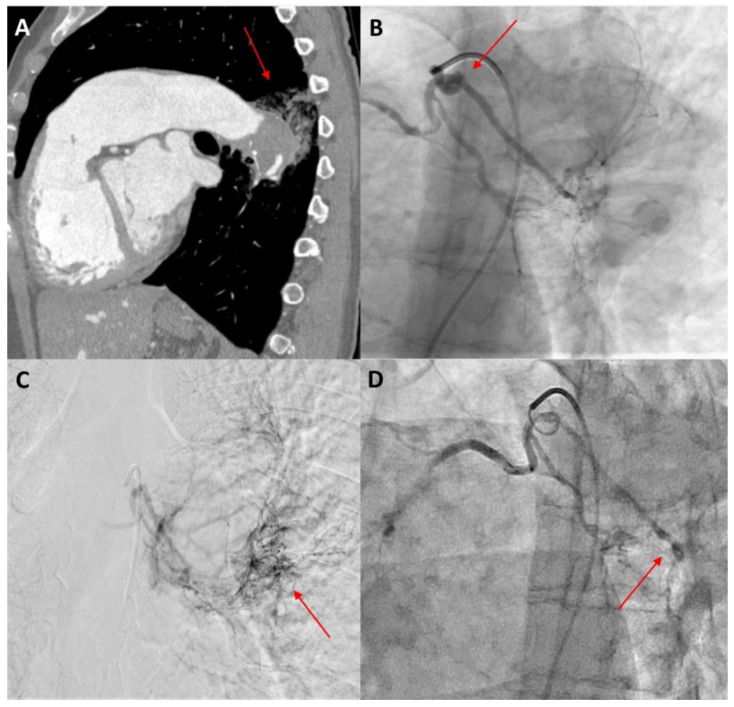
A 40-year-old female patient with Eisenmenger syndrome on the background of a large secundum ASD presenting with recurrent episodes of haemoptysis. (A) Sagittal view of thorax computed tomography after intravenous contrast medium injection, showing aneurysmatic dilatation of the left pulmonary artery with intraluminal thrombus and adjacent post-bleeding infiltrations in the lung parenchyma (red arrow). (B) Catheterization of the bronchial arteries with a 5 Fr Cobra catheter, showing the tortuous origin of the left bronchial artery (red arrow). (C) Angiographic image in a delayed parenchymal phase, showing abnormal imaging of the left posterior lung parenchyma adjacent to the descending aorta, corresponding to the area of bleeding (red arrow). (D) Angiographic image after embolization with 100–300 μ particles through a 2.6 Fr microcatheter. The left bronchial artery could not be selectively catheterized, so injection of particles was performed in both bronchial arteries until stasis was achieved, especially in the left side (red arrow).
